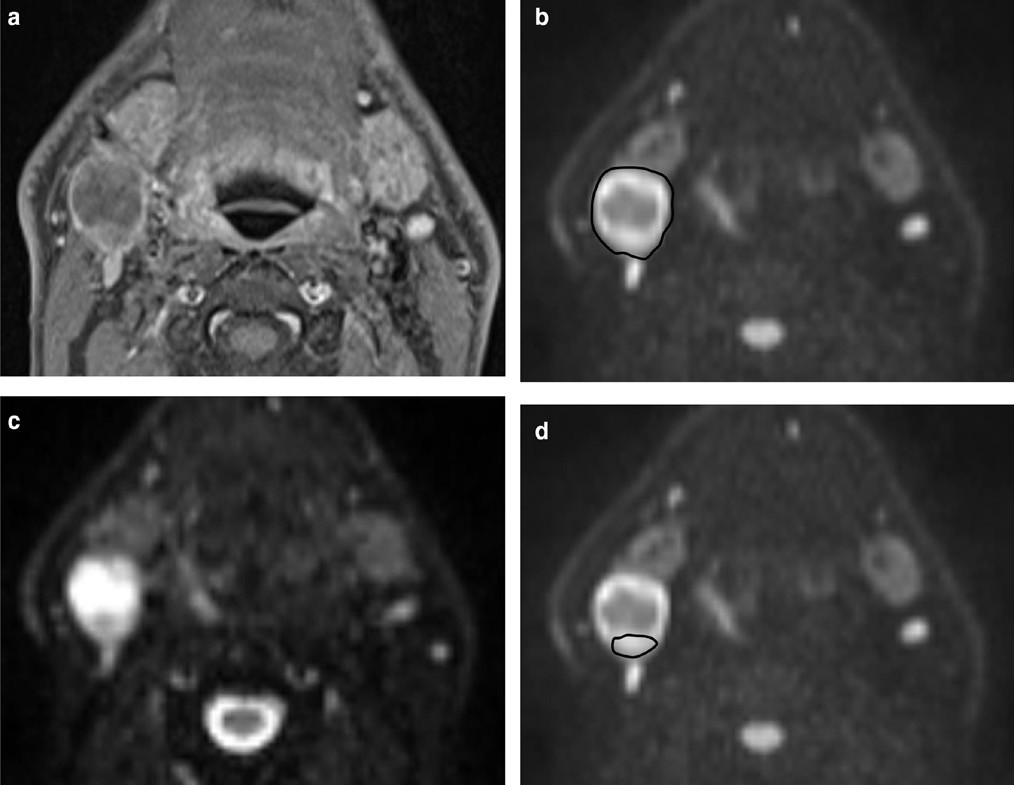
A HPV positive oropharyngeal cancer patient with a largely cystic right level two lymph node. (a) *T*_1_W post-gadolinium axial image b) *b* = 0 s/mm^2^ map from DW-MRI c) *b* = 800 s/mm^2^ map from DW-MRI indicating aROI as the whole of the lymph node outline and d) *b* = 800 s/mm^2^ map from DW-MRI indicating rROI as the component of the lesion returning intermediate signal on the *b* = 0 map and with enhancement on the *T*_1_W post gadolinium axial image. DW-MRI, diffusion-weighted MRI; HPV, human papilloma virus; ROI, region of interest.
